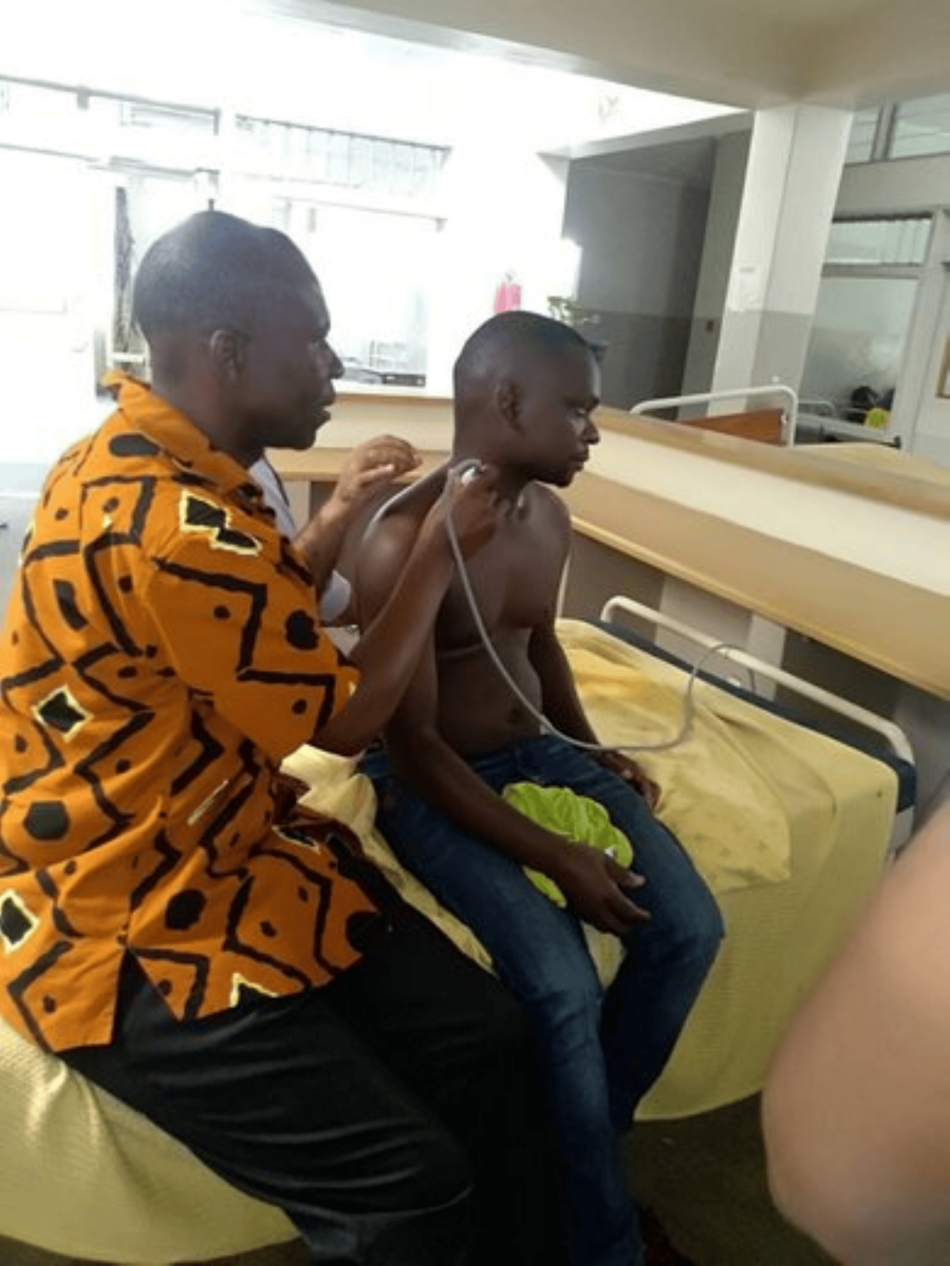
Two anesthesia healthcare officers practicing obtaining POCUS views on one another Consent was obtained from all healthcare workers shown POCUS, point-of-care ultrasound
